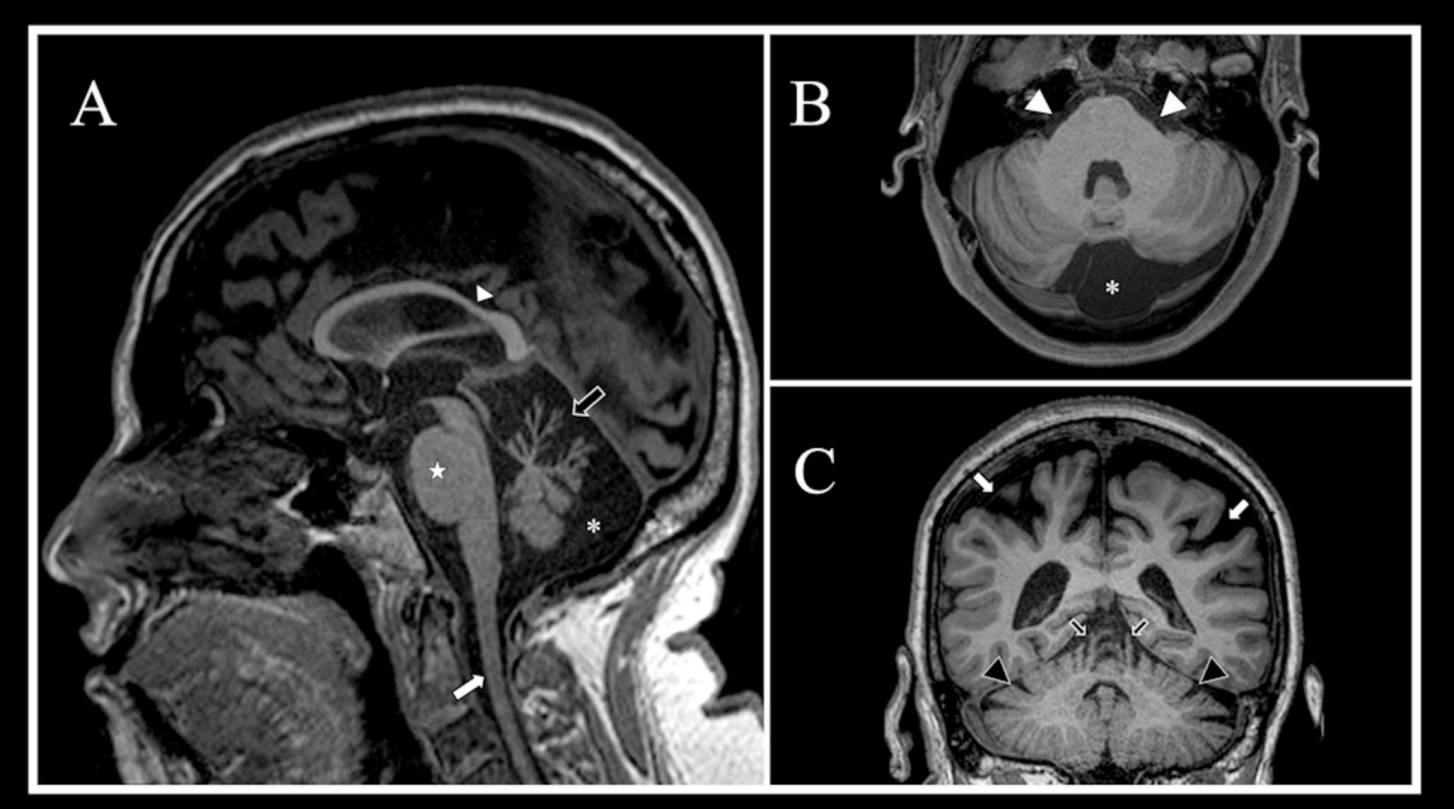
Patterns of atrophy detectable via conventional MRI in ARSACS. A sagittal midline T1-weighted image (A) showing in an ARSACS patient the typical occurrence of superior vermis atrophy (*black arrow*) along with a “bulky” appearance of the pons (*white star*). Other imaging findings include a thinning of the mid-posterior segment of corpus callosum (*white arrowhead*), a mild atrophy of the upper cervical cord (*white arrow*) and the evidence of a posterior fossa arachnoid cyst (*white asterisk*), with the latter finding also visible in the axial multiplanar reconstruction in (B), where is also possible to appreciate the thickening of middle cerebellar peduncles (*white arrowheads*). Finally, a coronal multiplanar reconstruction (C) confirming the occurrence of a significant superior vermis atrophy (*black arrow*s), coupled to a mild degree of cerebellar hemispheres (*black arrowheads*) and, more pronounced, biparietal (*white arrows*) atrophy
